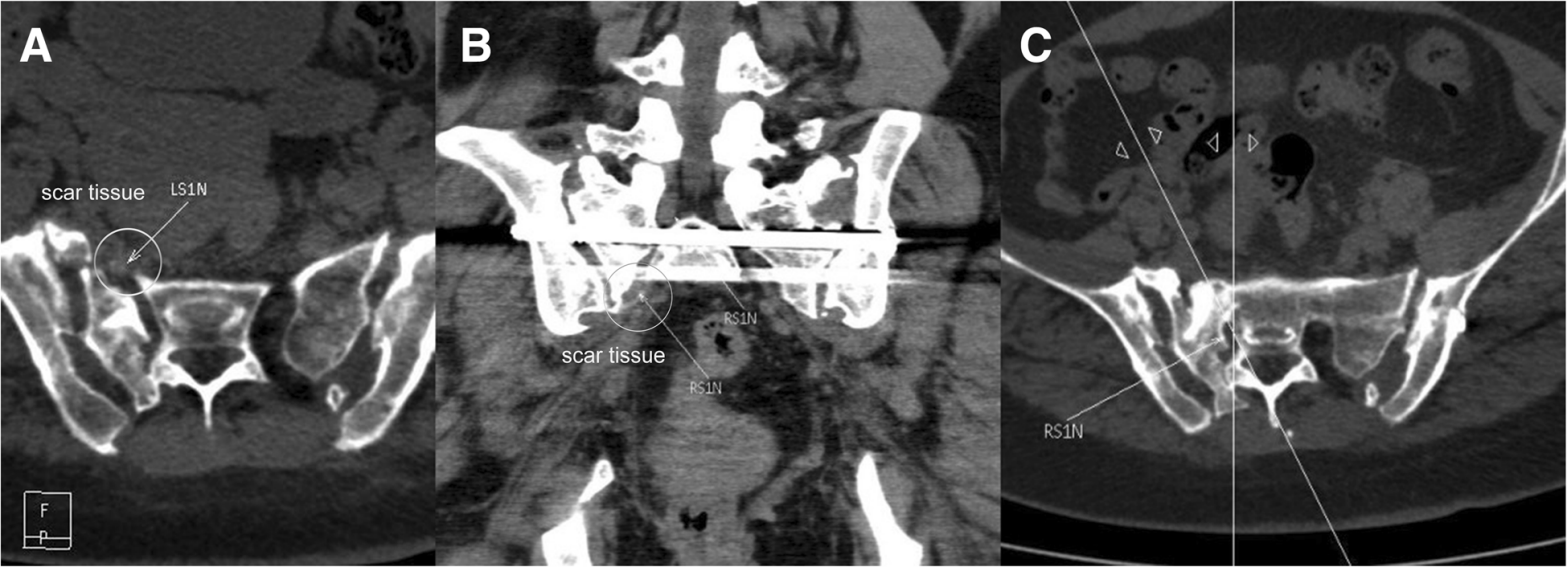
a–c The multiplanar CT reconstruction of sacral nerve showed the scar tissue (white circle) and S1 nerve (white arrow)
